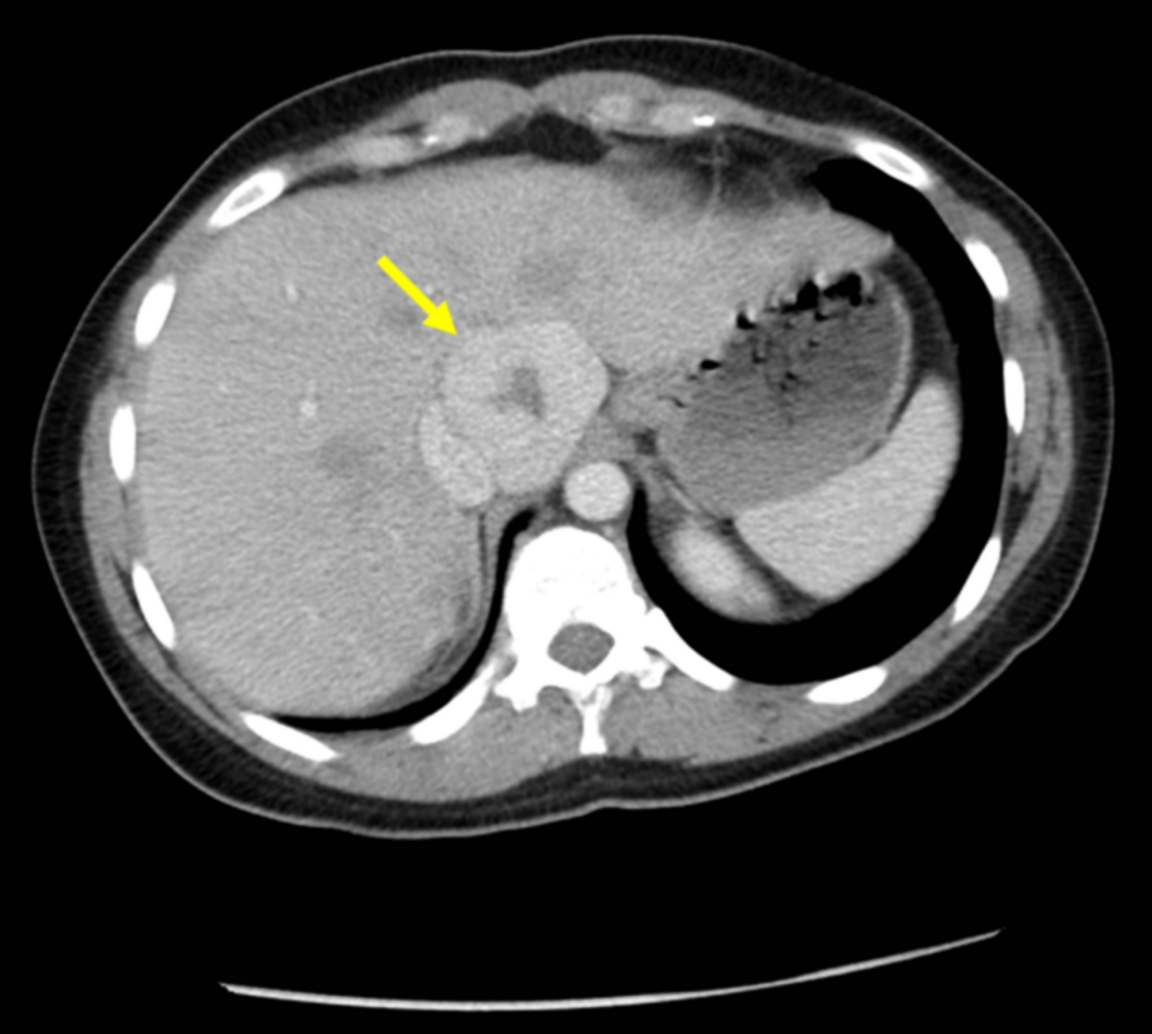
Contrast-enhanced abdominal CT CT: computed tomography Contrast-enhanced CT in portal venous phase demonstrates an enhancing mass with central non-enhancement. Imaging characteristics favor focal nodular hyperplasia
